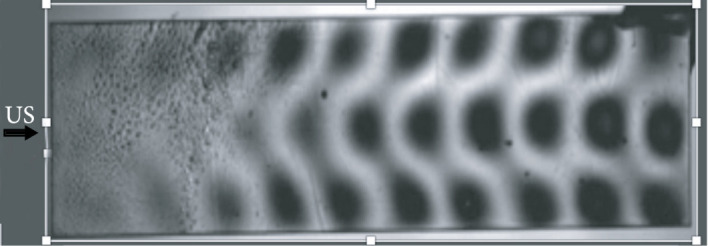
Photoelastic image of stress waves created in a model stone by BWL. Photoelastic imaging reveals the stress (black) of the guided waves in the 5×15 mm acrylic bar. The arrow shows the direction of ultrasound propagation (350 kHz). In this larger stone, the multiple-cycle structure of the BWL pulse causes guided waves to develop which cyclically concentrates and amplifies the stress [14] (courtesy of Adam Maxwell, University of Washington).
